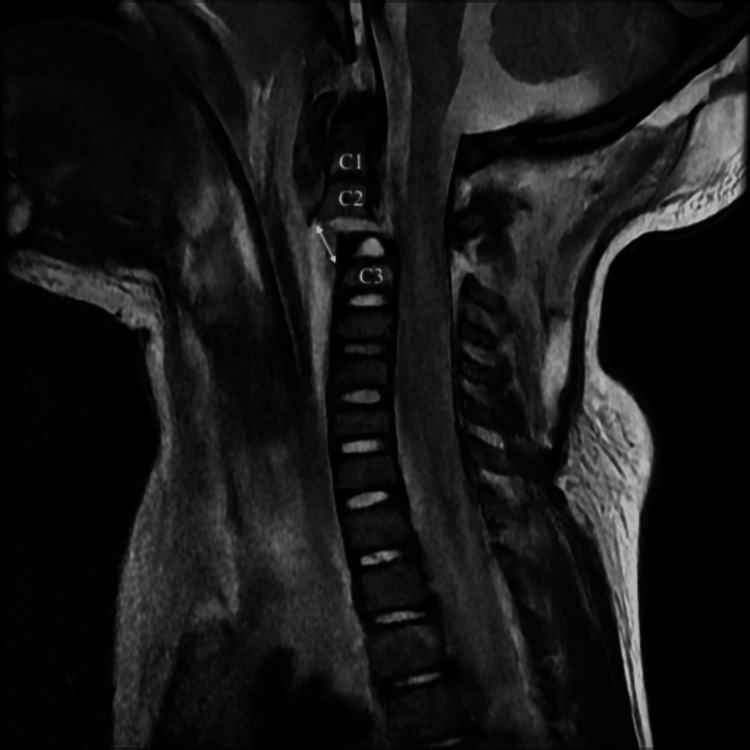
Sagittal T2 C-spine magnetic resonance imaging (MRI) demonstrating avulsion fracture at the base of C2 and malalignment of cervical spine.
